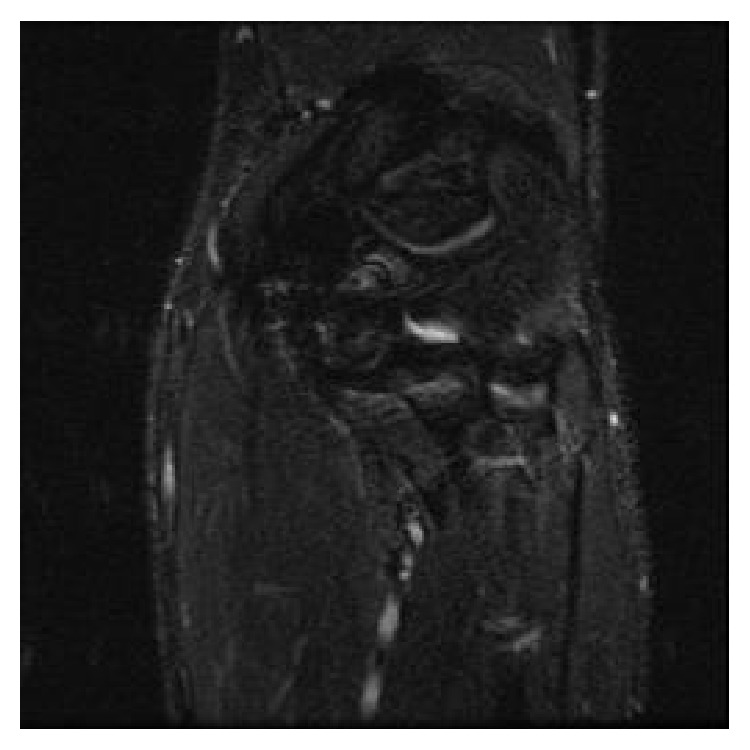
17-month postoperative radiograph. Coronal STIR MRI demonstrates postoperative changes and the medial aspect of the elbow. There are intact low signal intensity fibers extending from the medial epicondyle to the sublime tubercle of the proximal ulna, without retraction or deformity at the reconstruction site.
